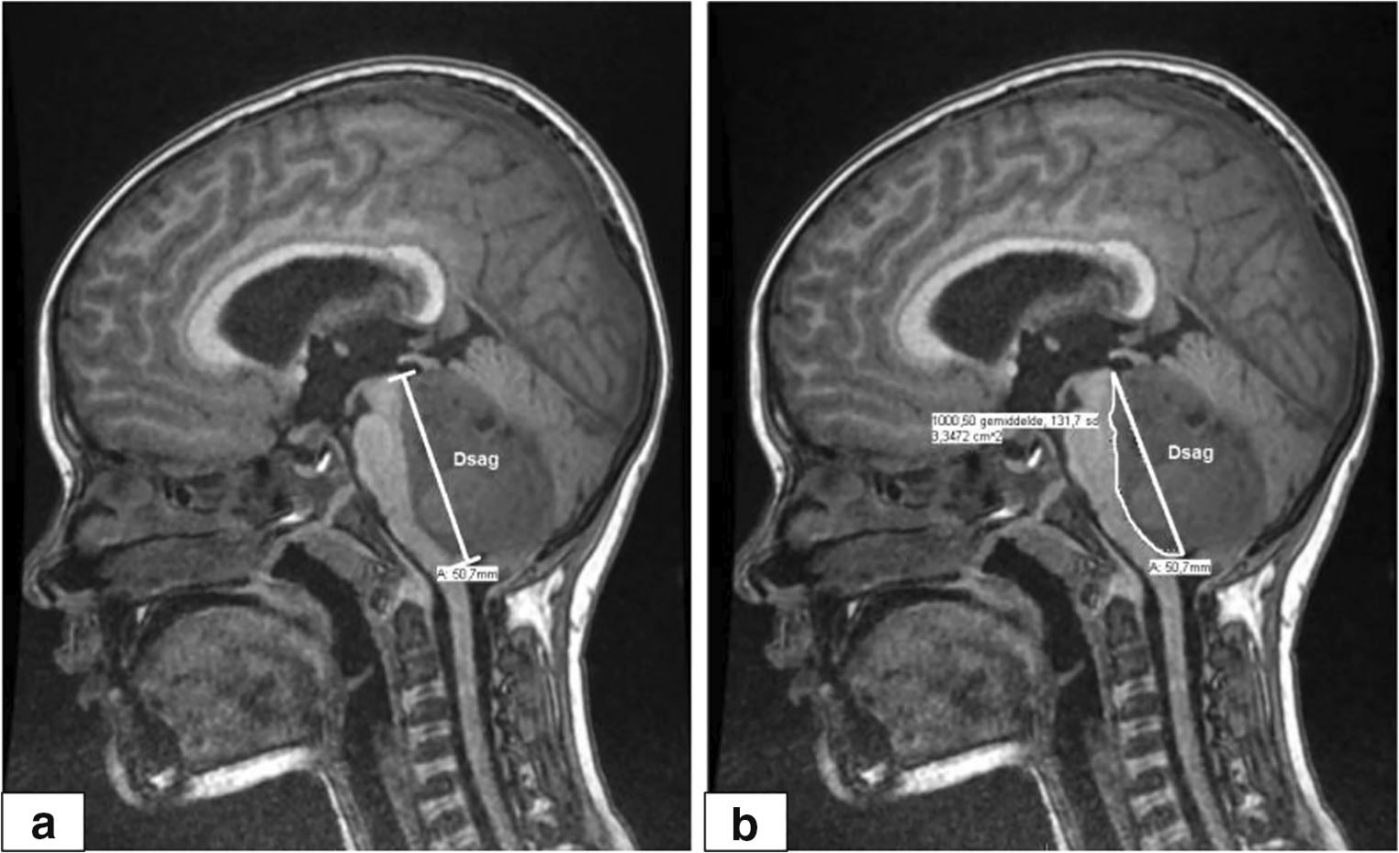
Example of measurement of area: A(sagittal): using the D(sagittal) line in the midline as reference (a), the outlines of the tumor that invaded and/or compressed the brainstem were traced (b). The estimated size of the area of invasion/compression was calculated by the program used to evaluate the MRI-scans
